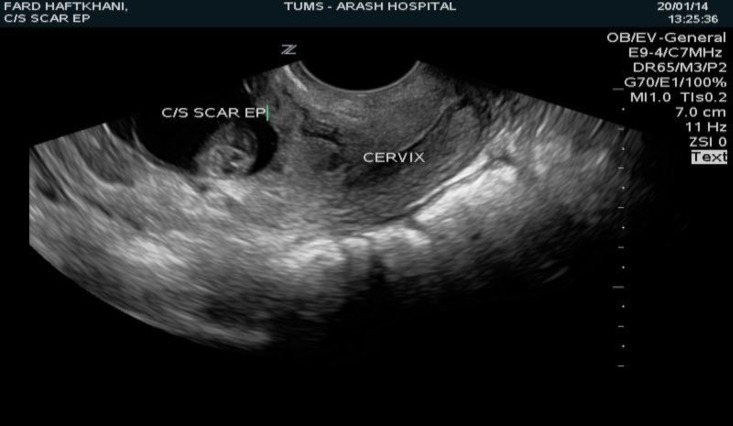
Cesarean scar pregnancy demonstrated by transvaginal sonography showing the gestational sac implanted in the lower segment of the uterus
